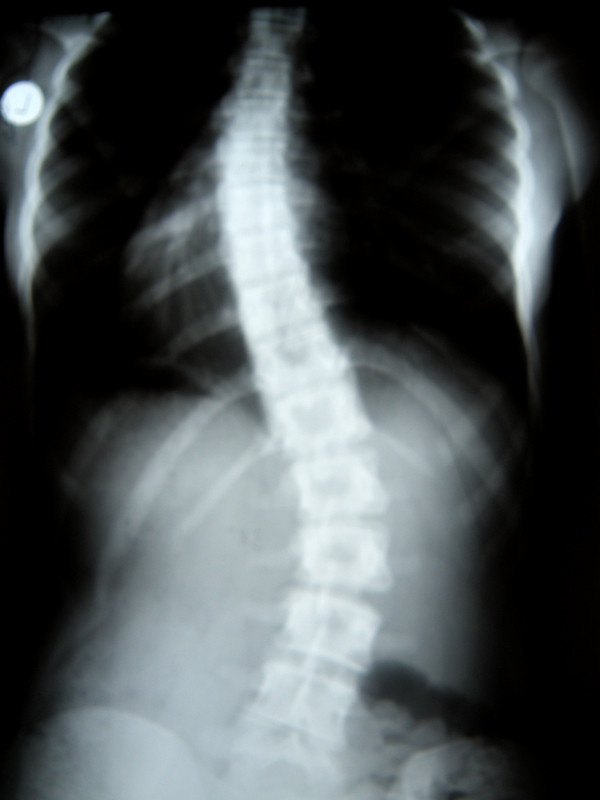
Radiography before treatment.
